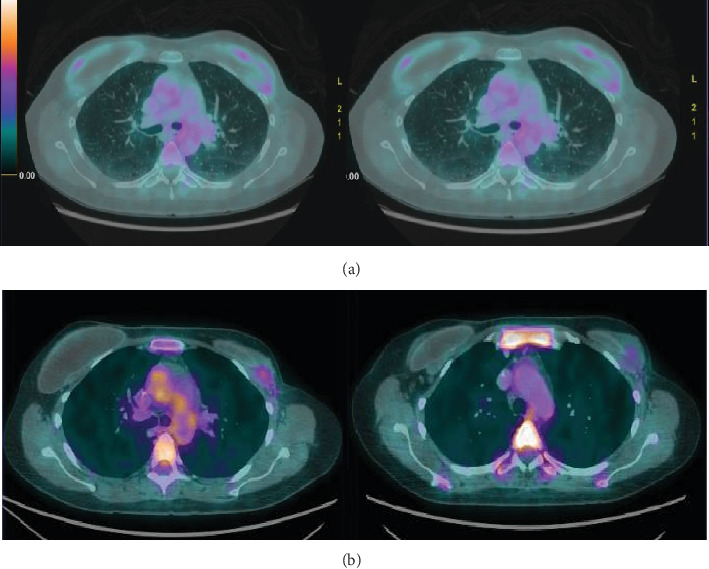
PET-CT scan during systemic treatment shows reduction of nodules and absence of contrast uptake. (a) Before starting chemotherapy. (b) After six cycles of chemotherapy.
